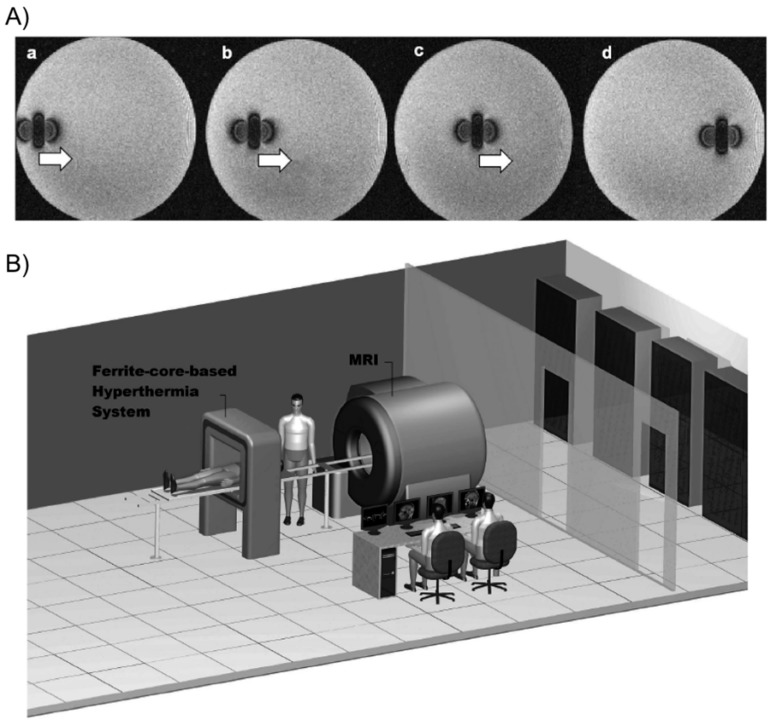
(A) MRI images of the microrobot at four different positions. The artifact caused by the microrobot approximates the magnetic dipole field that it induces, making it possible to track its position; and (B) overview of the magnetic resonance tracking and guiding platform integrated with the magnetic hyperthermia system. The patient can easily be transferred from one system to another for a complete delivery procedure. Adapted with permission from reference [66]. Copyright ^©^ 2011 Taylor & Francis.
